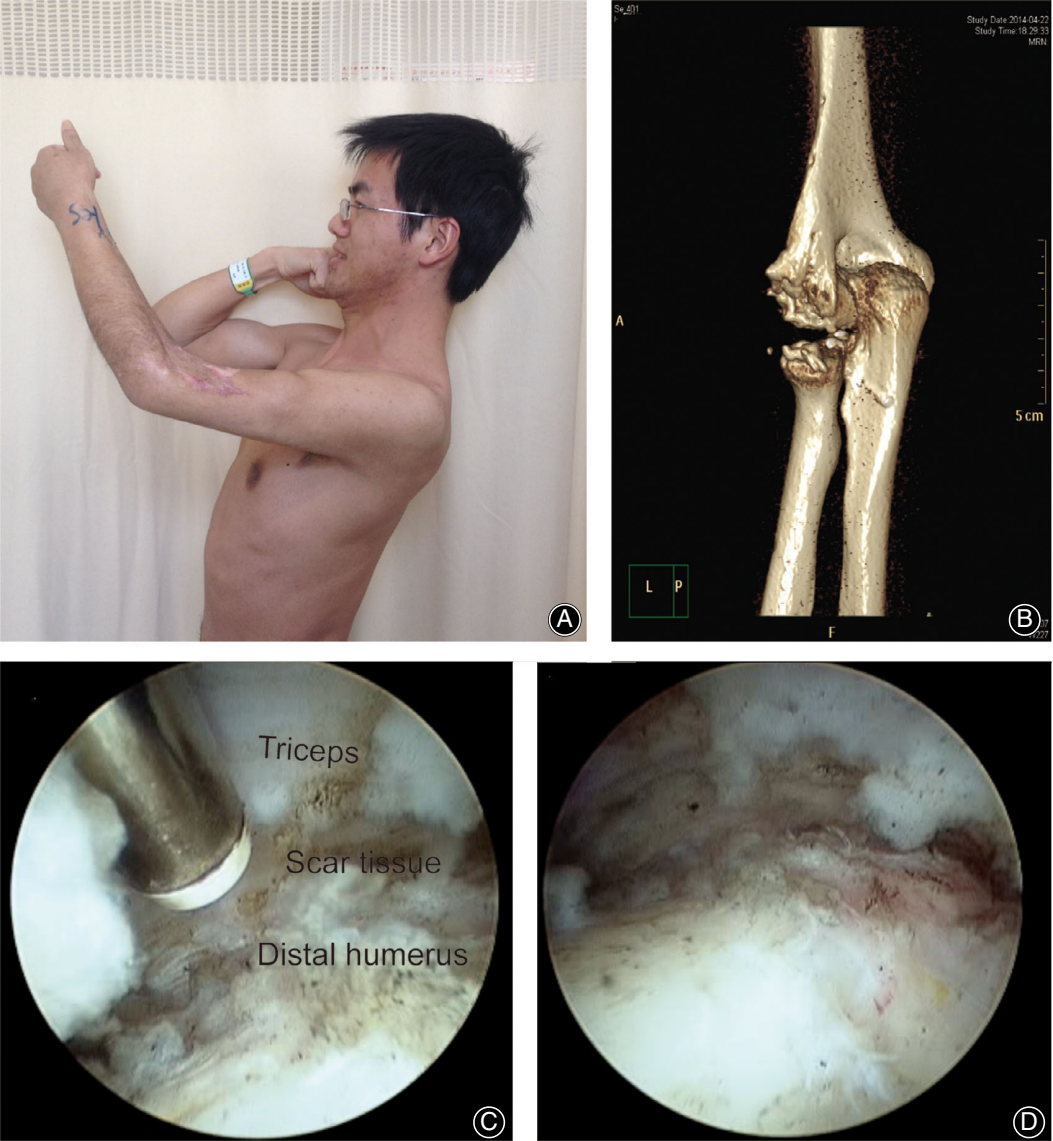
Posttraumatic stiff elbow in a 21‐year‐old man. (A) Preoperative physical examination shows very limited elbow flexion. (B) CT scan shows heterotopic ossification (HO) formation after previous surgery. (C, D) Radiofrequency was used to release muscle from the distal humerus.
